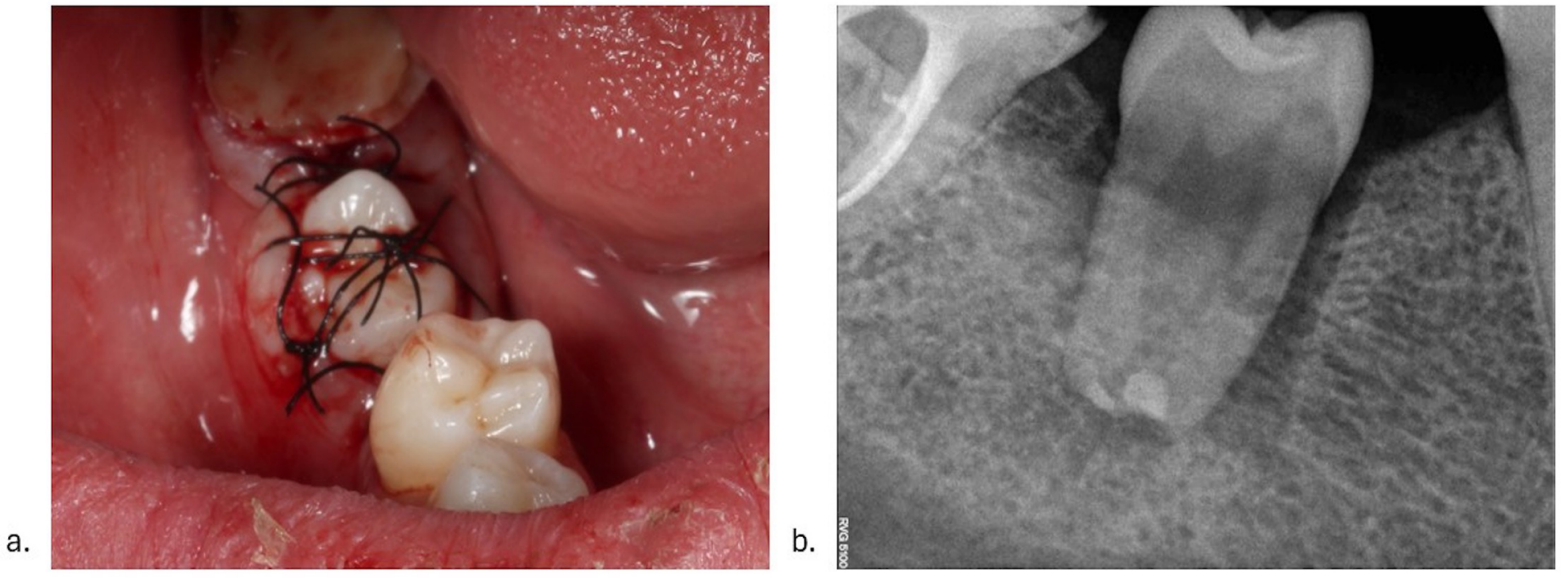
Intraoral aspect following tooth autotransplantation of tooth 25 in the position of 46. (a) Postoperative view of the autotransplanted tooth. Suture stabilization was acquired. (b) Postoperative radiograph of the autotransplanted tooth, showcasing a loose fit.
